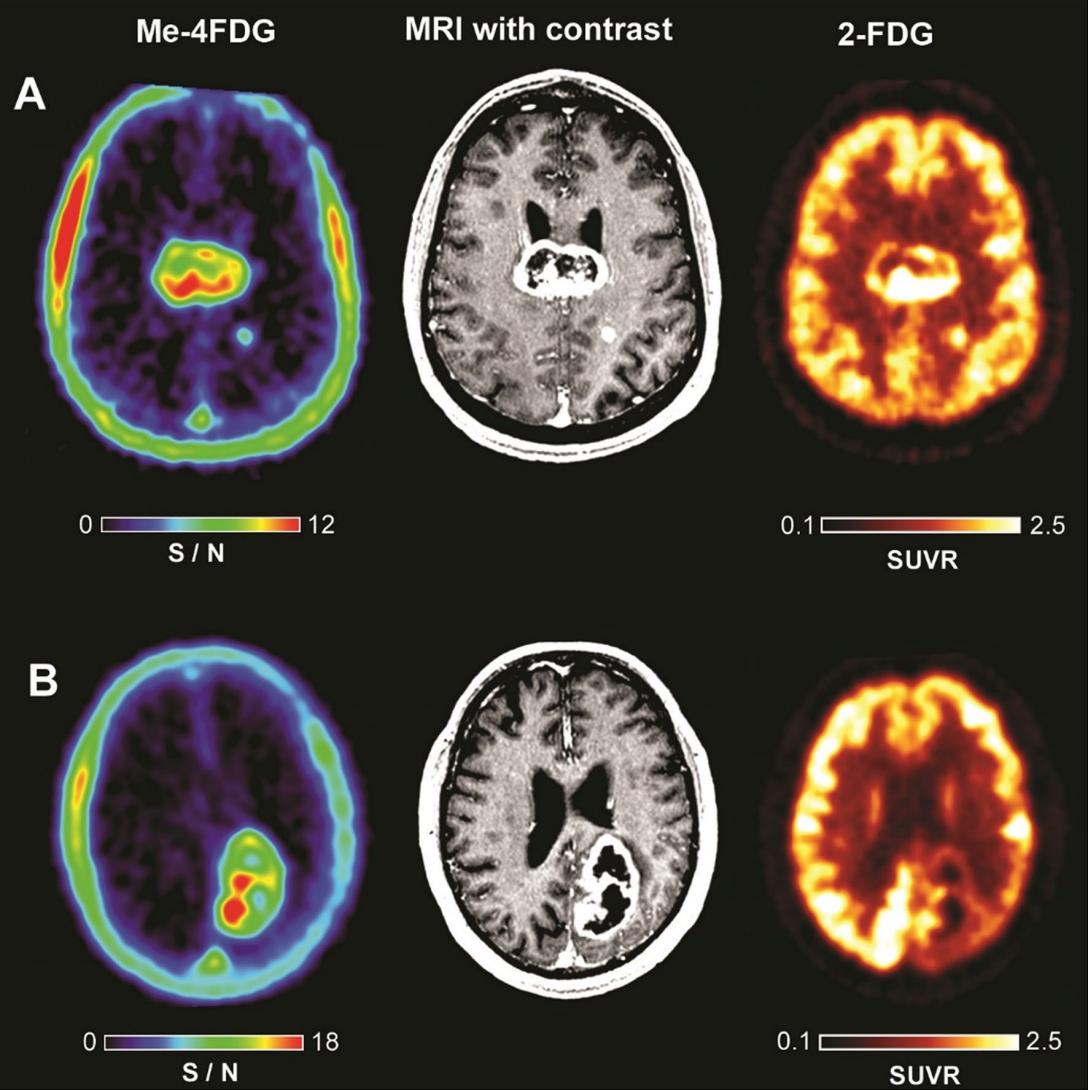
Me-4FDG PET, 2-FDG PET, and MRI scans on WHO Grade IV astrocytoma patients. a Patient with a 46-mm posterior corpus callosum astrocytoma, and b patient with 53-mm posterior para median left parietal lobe glioblastoma. For Me-4FDG the S/N (SUVR/BG) the NIH color scales are those relative to the torcula, and for 2-FDG the SUVR scales are for the “Hot-Iron” color scale. SUVR for 2-FDG was based on white matter as reference region and not blood. SUVR for Me-4FDG was based on blood activity and we re-normalized it to brain tissue so that we actually are comparing the same values. Thus, that is why S/N is specified for Me-4FDG and SUVR is specified for 2-FDG in the scale bar
